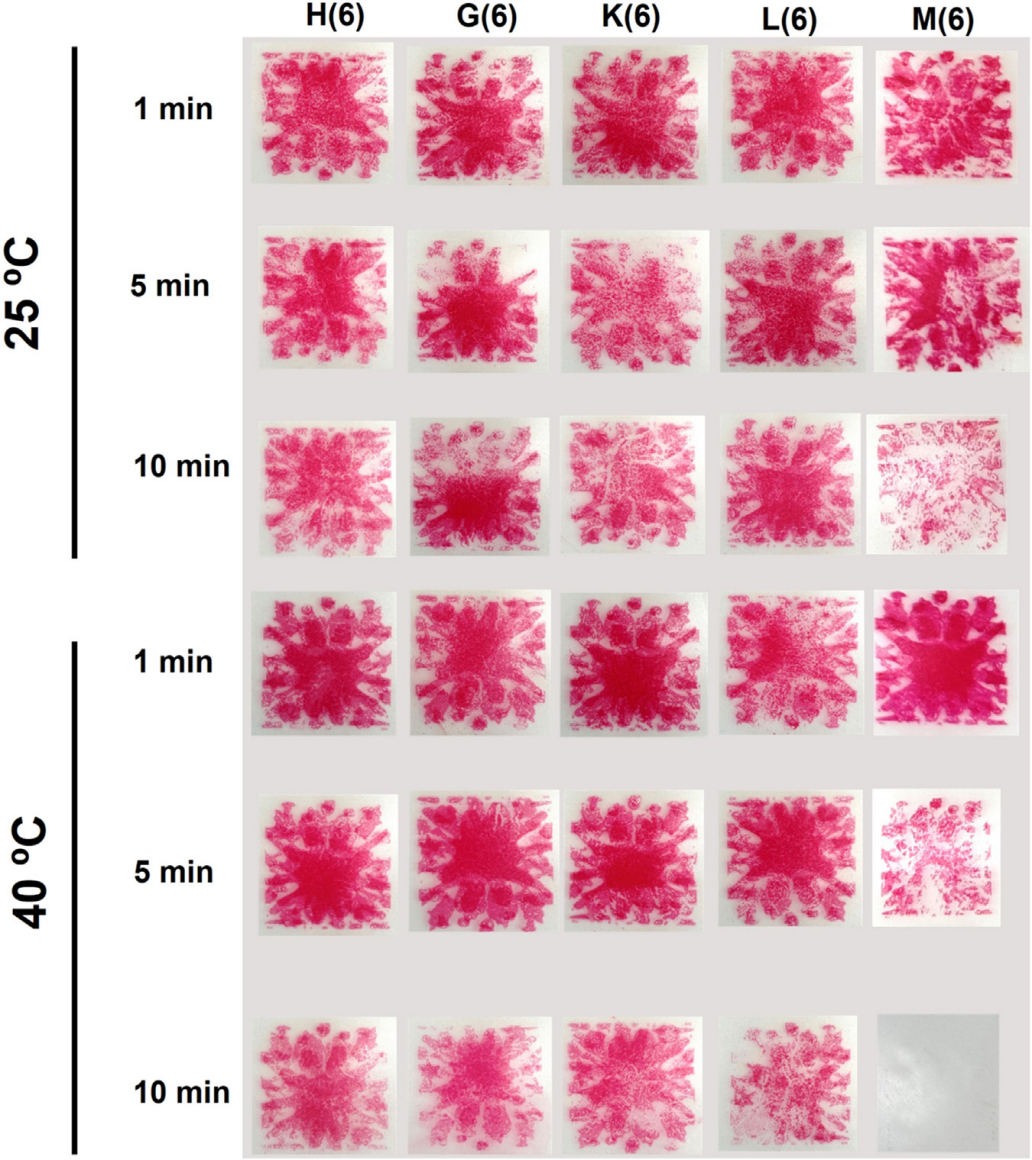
Effect of temperature and time on ultrasonic cleaning efficacy for products used at 6 mL/L in tap water (number within brackets indicates the concentration used in mL/L).
